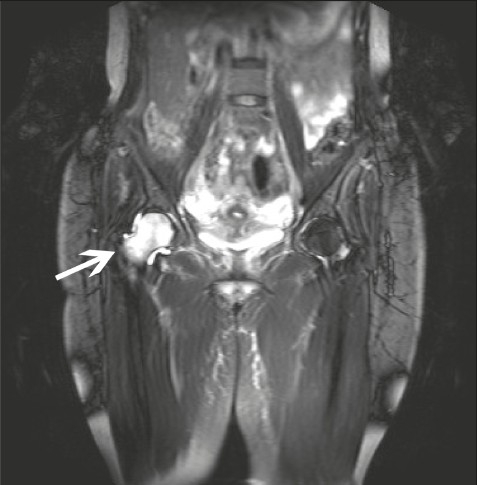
Initial MRI with the classical bone marrow edema picture of the right femoral head and neck.
